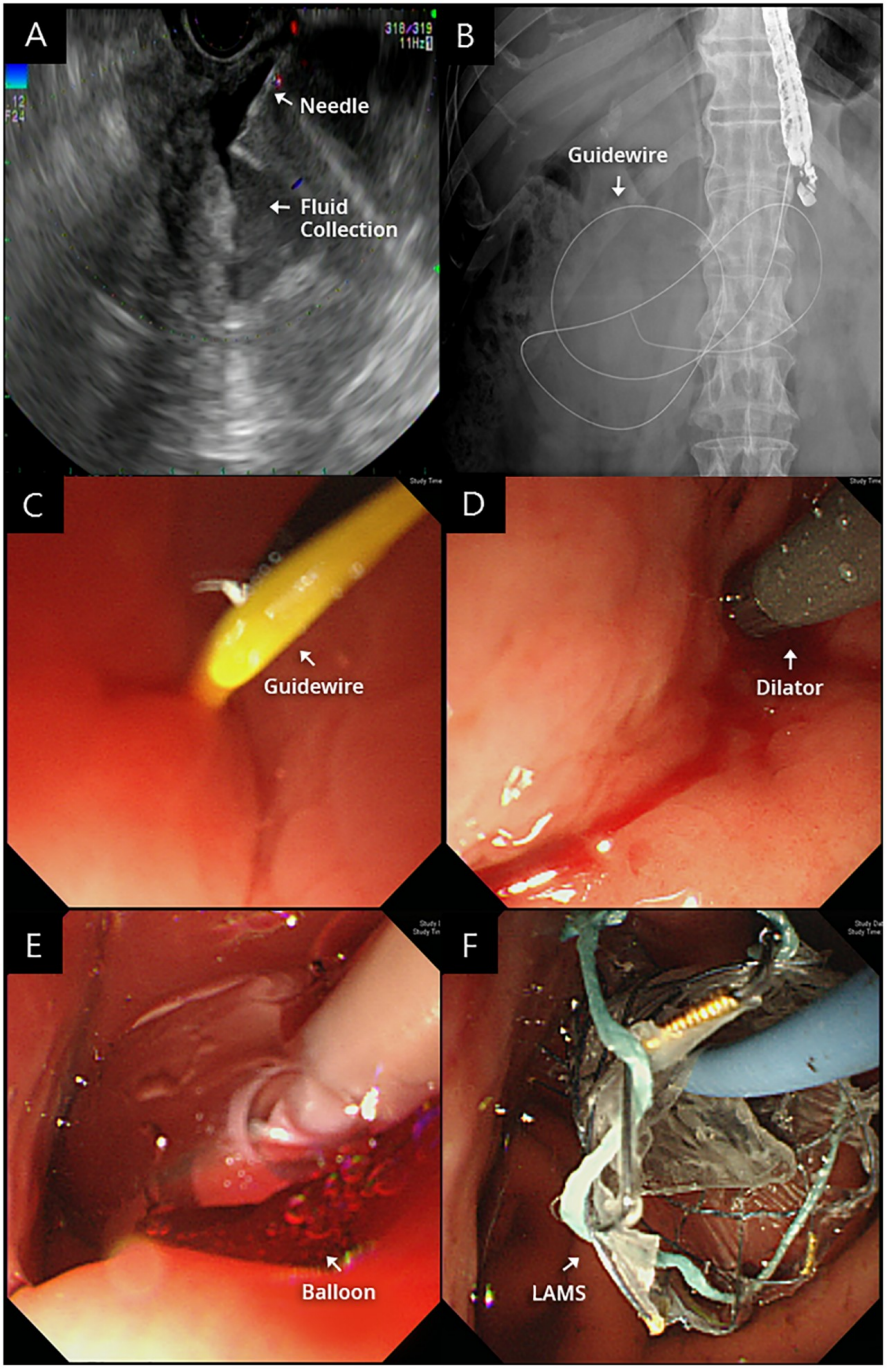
Endoscopic ultrasound (EUS)-guided pseudocyst drainage using the lumen-apposing metal stent (LAMS). (A) The lesion was punctured under EUS (Doppler) guidance with a 19-gauge EchoTip needle (B). (C) A guidewire was inserted in the lesion. (D) The puncture site was dilated using a Soehendra dilator. (E) Balloon dilatation using a Hurricane balloon. (F) The LAMS was deployed through the scope.
